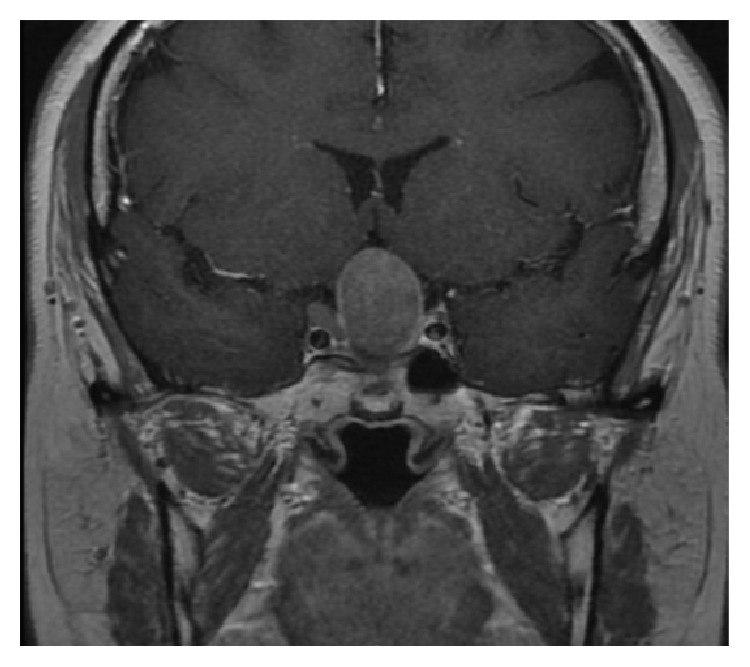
Coronal MRI T1 sequence with gadolinium. Intra- and suprasellar tumor with extension to the floor of third ventricle, after first surgery.
